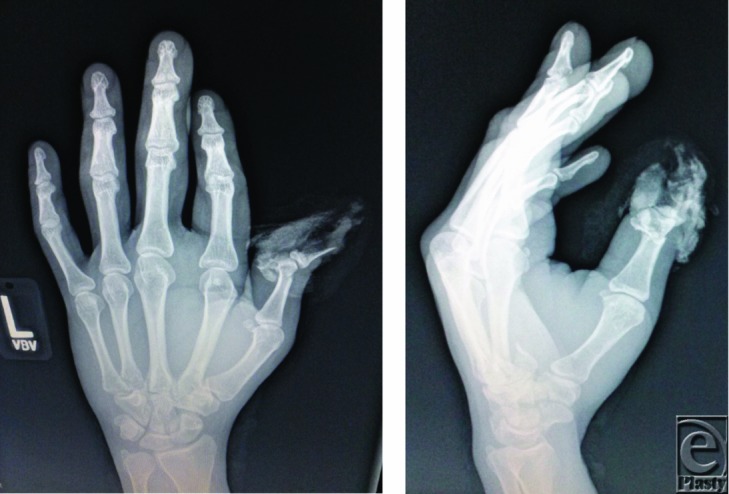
Radiographs of the distal thumb crush-avulsion revealing distal phalanx bone fragments and proximal phalanx transverse fracture.
